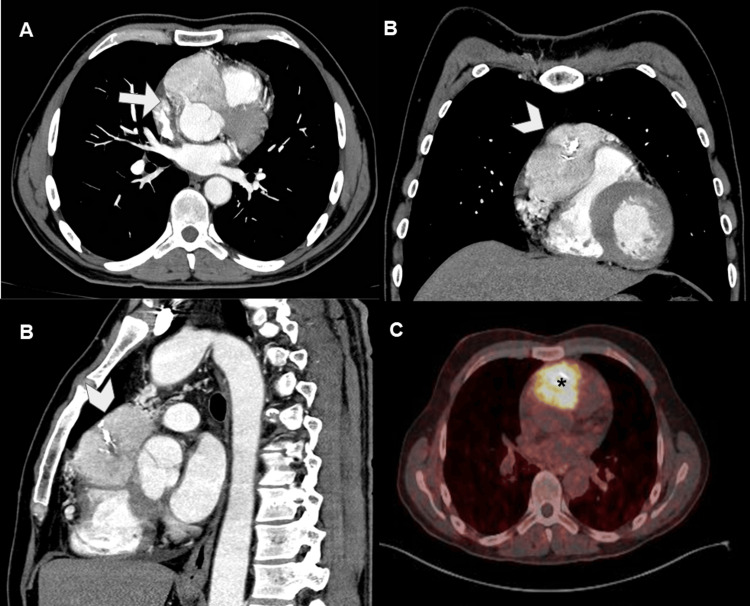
Cardiac paraganglioma in the right atrioventricular sulcus. CECT on axial, coronal, and sagittal reconstructions (A-C) and PET-CT with somatostatin analogue (C). The images show a hyperenhancing mass in the right atrioventricular sulcus and occupying the anterior mediastinum. In the axial view, the mass surrounds the origin of the right coronary artery (arrow in A). Coronal and sagittal views show calcifications within the tumor (arrowhead in B and C). PET-CT shows uptake of the radiotracer by the tumor, demonstrating its neuroendocrine origin (asterisk in D). CECT: contrast-enhanced computed tomography; PET-CT: positron emission tomography-computed tomography.
